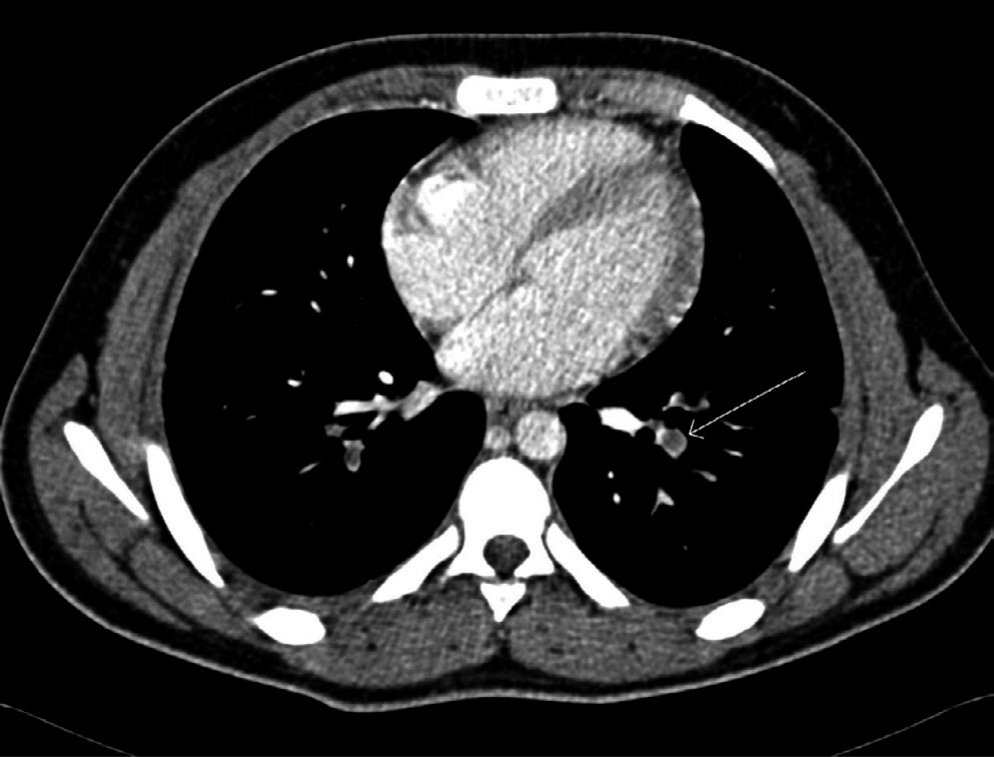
CT chest showed left side pulmonary embolism
